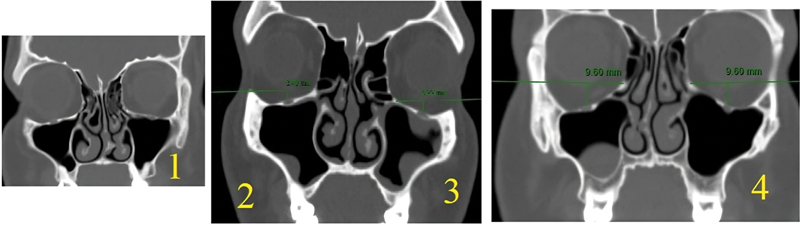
A CT scan of the different grades of the maxillary sinus roof.
